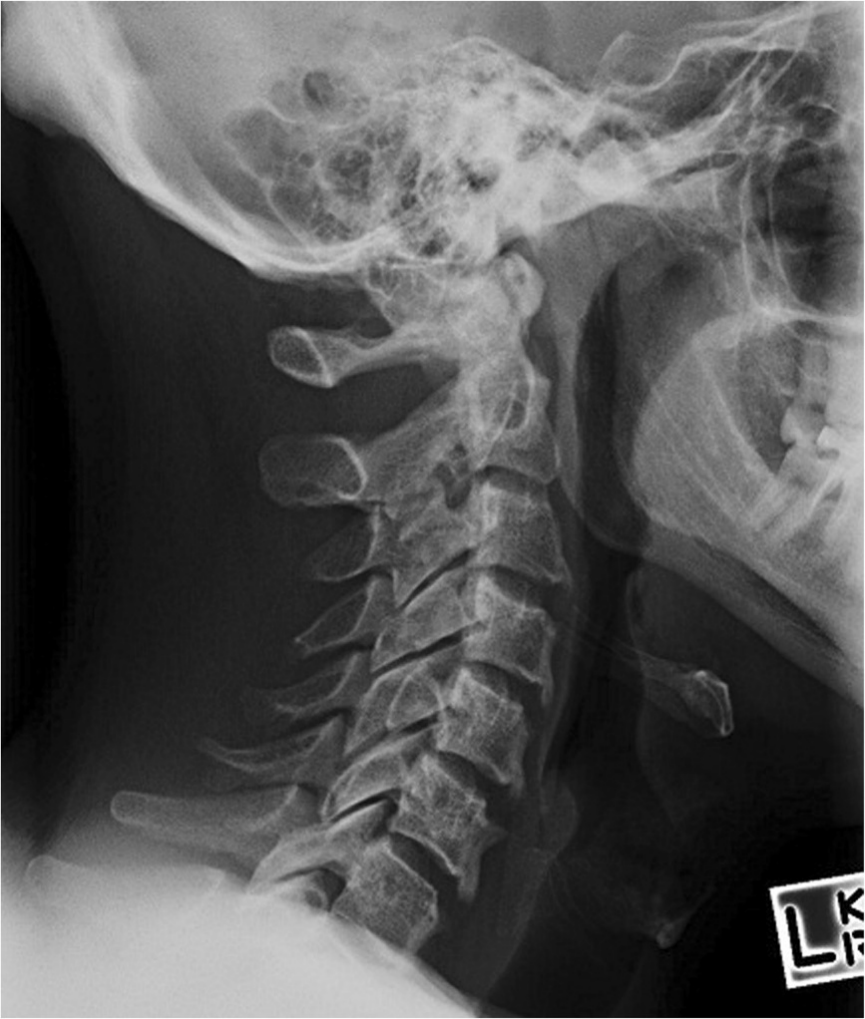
Lateral cervical spine X-RAY showing inferiorly-facing ossification along the C3-6 vertebral bodies.
